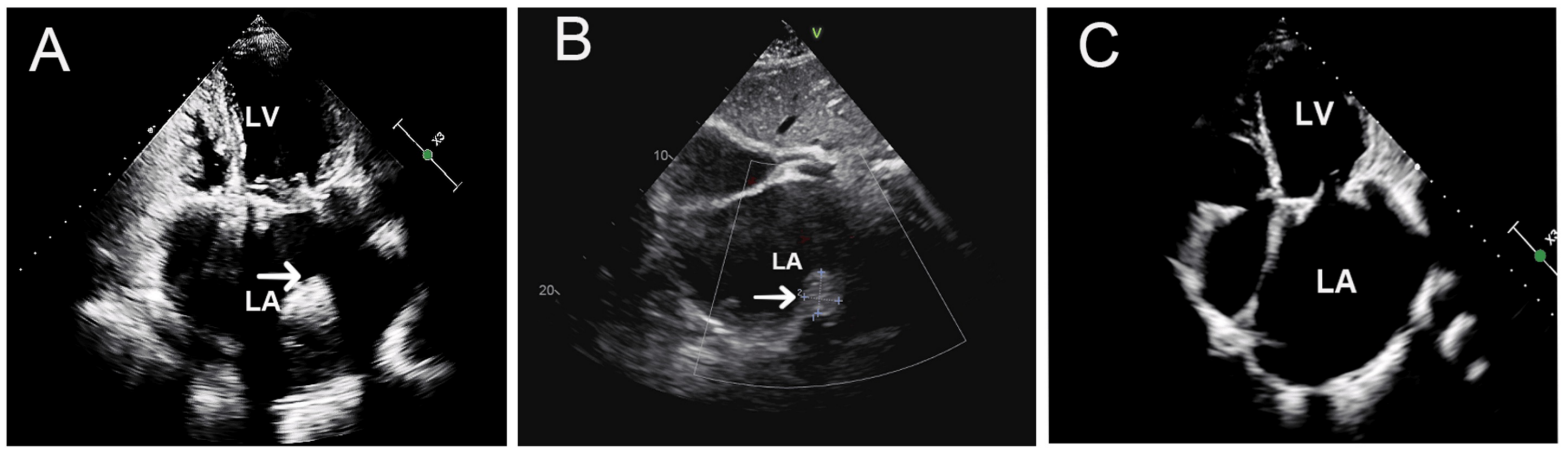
Transthoracic echocardiography showed the history of left atrial (LA) thrombus. Giant LA thrombus at baseline (arrow, A), with reduction after three weeks (arrow, B), and complete resolution after three months (C). LV: left ventricle.
